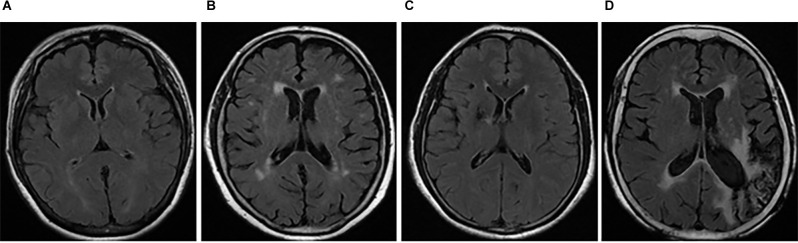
Typical WMH images in patients with CSVD and stroke. T2WI-FLAIR MRI sequences were analyzed in the patients with CSVD and/or stroke. The total Fazekas scores of typical patients with CSVD were 1 (A) and 4 (B), and those of typical stroke patients with infarction of the right basal ganglia and left occipital lobe were 1 (C) and 4 (D), respectively. Panels (A,C) were identified as mWMH, and (B,D) as sWMH. CSVD, cerebral small vessel disease; mWMH, mild white matter hyperintensities; NC, normal cognition; sWMH, severe white matter hyperintensities.
